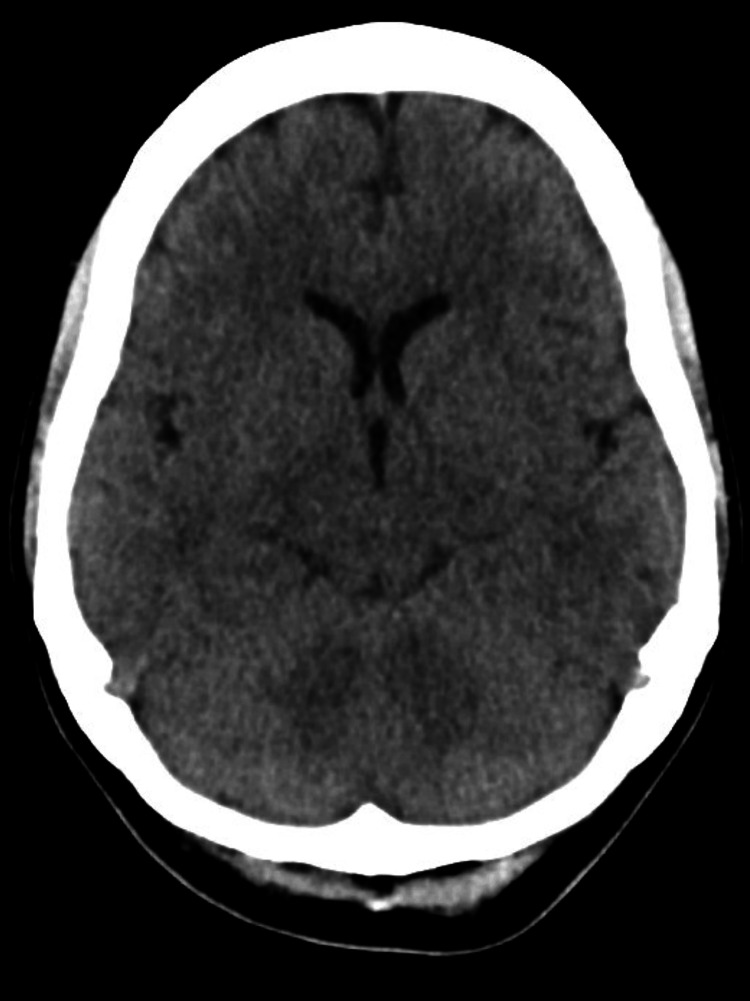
Normal axial CT brain showing no acute intracranial abnormality Axial non-contrast CT scan of the brain showing preserved gray-white differentiation and no evidence of hemorrhage, infarction, or mass effect.
